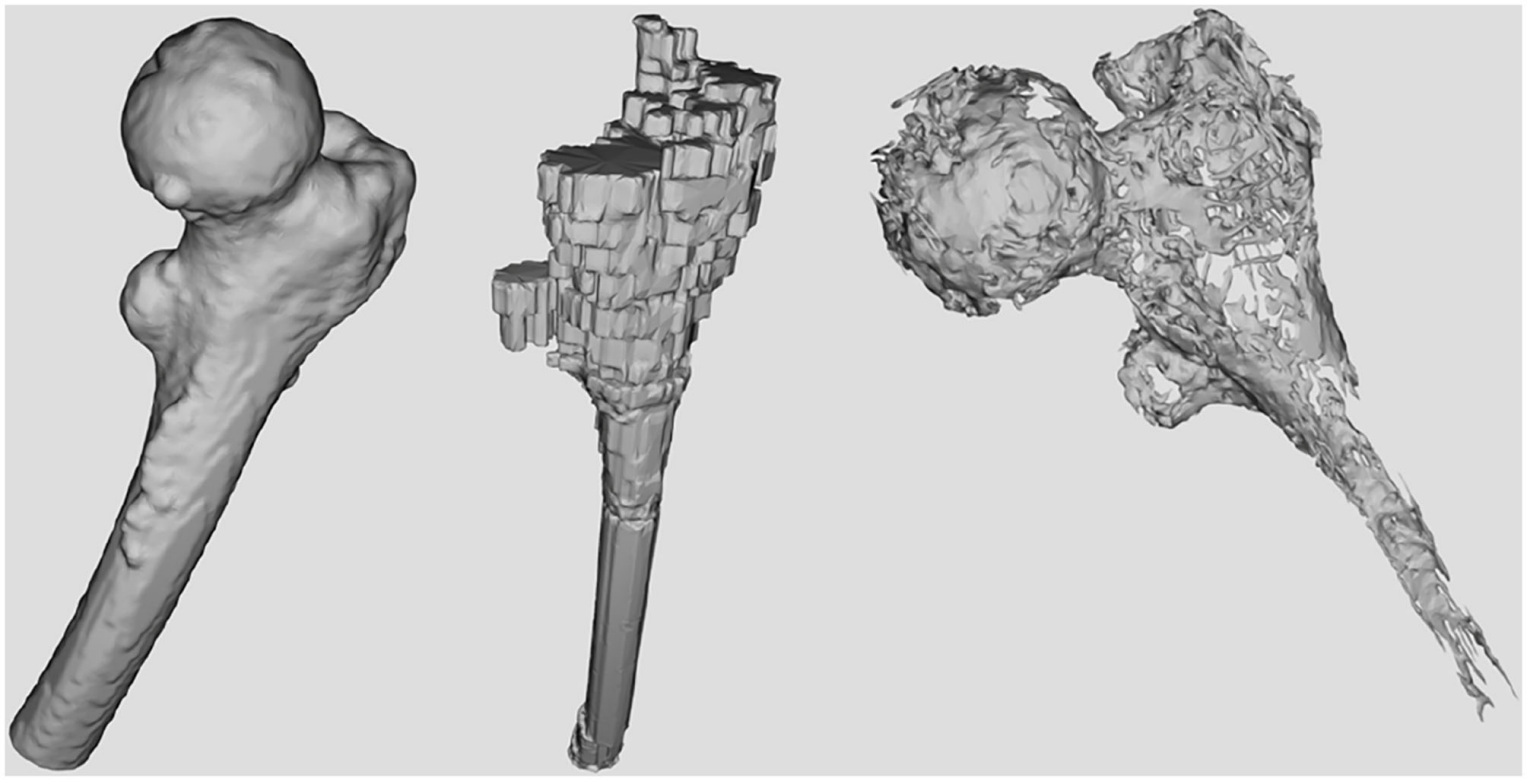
The surfaces corresponding to the bone's layers extracted from CT data. From left to right: Cortical bone surface, bone marrow, and necrosis.
